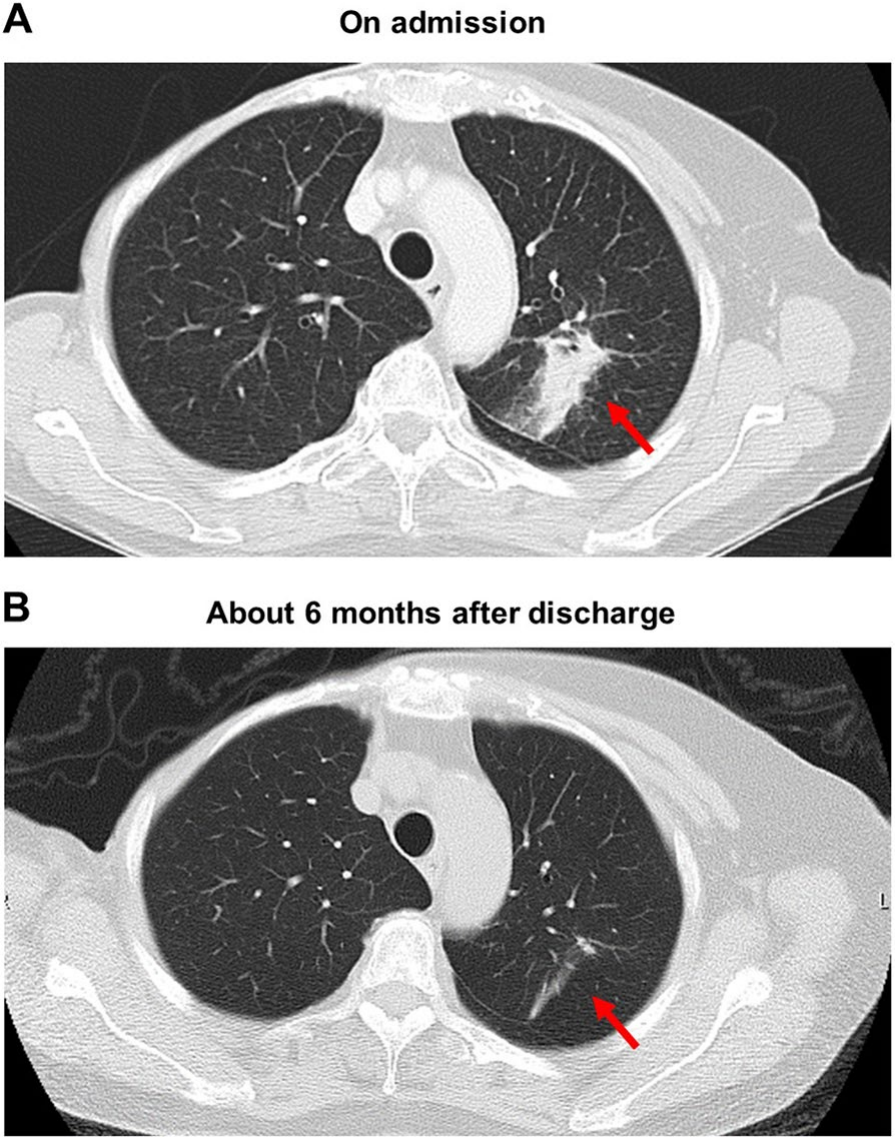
A A chest CT on admission showed marked consolidation in the upper lobe of the left lung. B A chest CT taken about 6 months later showed drastic reduction of the consolidation in the left lung compared to that on admission
